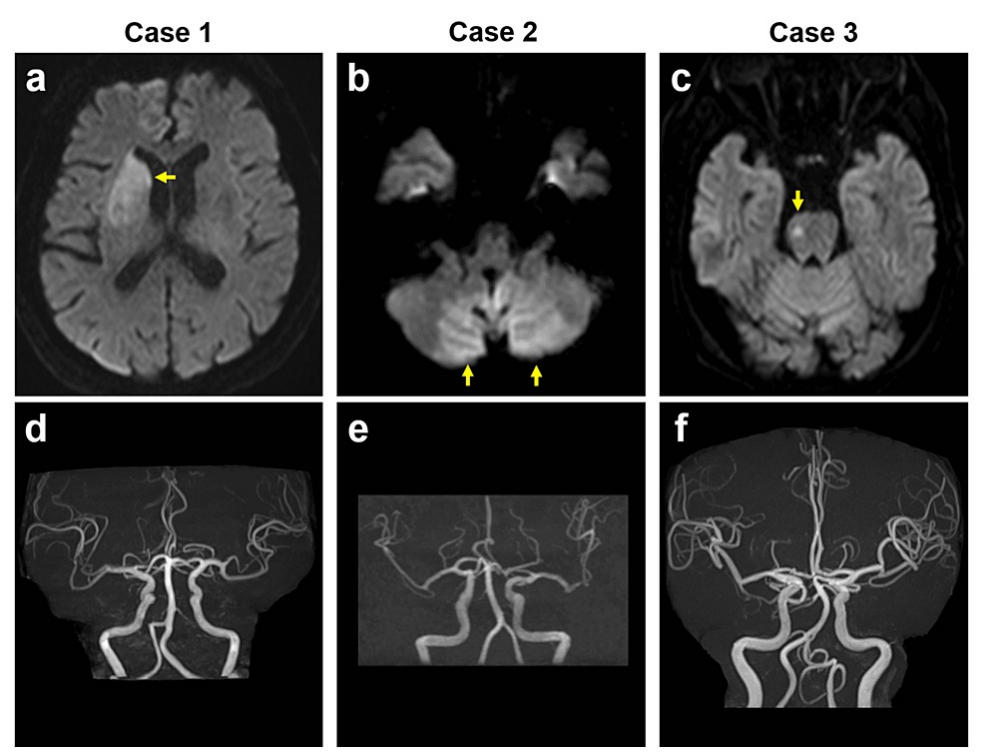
Brain MRI. Axial diffusion-weighted images showing acute infarction in the right basal ganglia due to embolism of the lateral striatum arteries in Case 1 (a, arrow), in both cerebellar hemispheres due to embolism of the posterior inferior cerebellar arteries in Case 2 (b, arrows), and in the right pons due to small-vessel occlusion in Case 3 (c, arrow). MRI angiography showing no occlusions of major cerebral arteries in Case 1 (d), Case 2 (e), or Case 3 (f). MRI, magnetic resonance imaging
